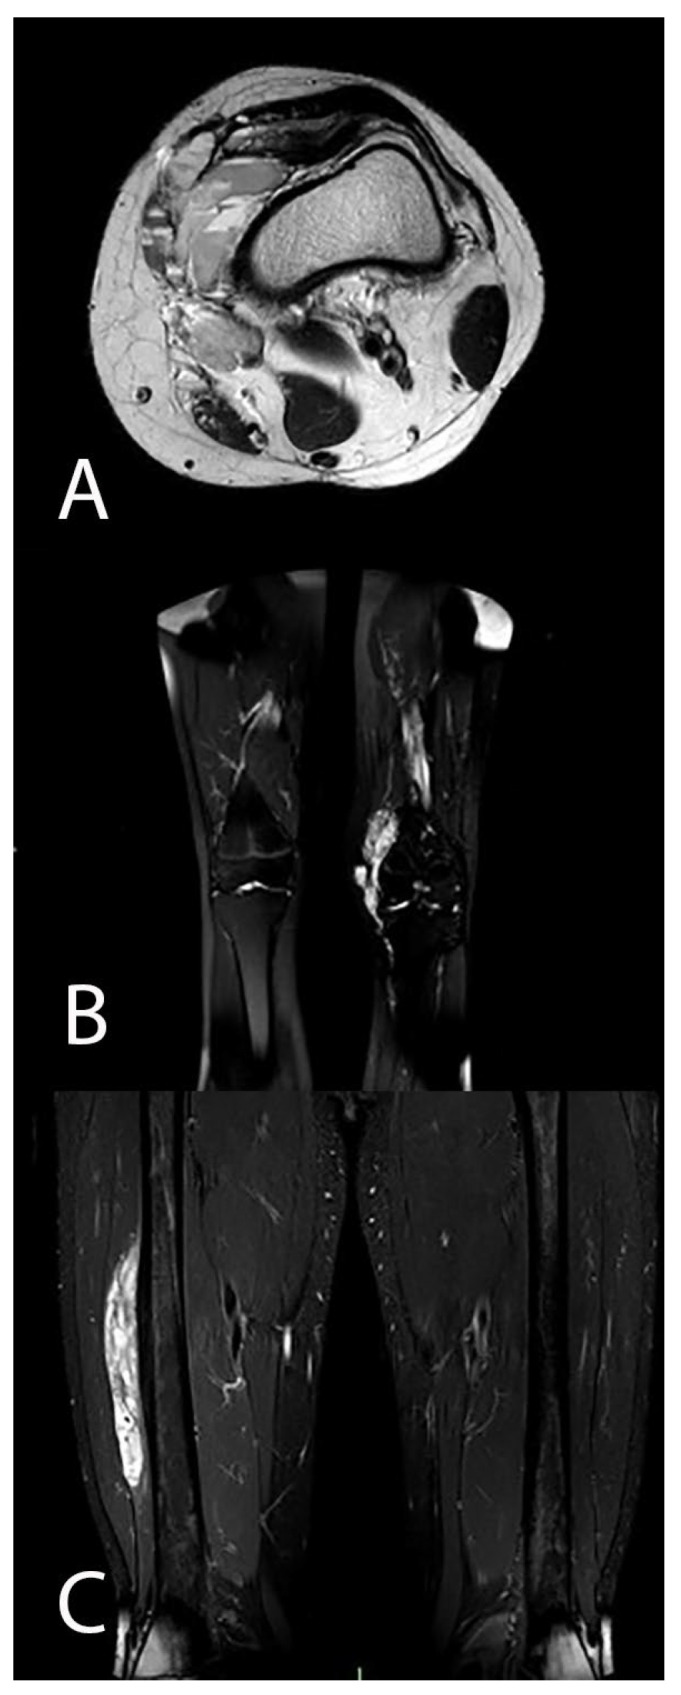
(A). Axial view T2 sequence MRI showing increased signal of a soft tissue MAV near the medial region of the knee, showing some fluid–fluid level related to possible hemorrhage or high protein content. (B). Coronal view T2 fat sat sequence MRI demonstrating hyperintensity of the signal of a soft tissue venous malformation in the medial region of the knee, with some intralesional low-intensity septa. T2 sequences help define the extent of the lesion. (C). Coronal view STIR sequence MRI shows high hyperintensity of the signal of an intramuscular venous malformation adjacent to the femoral diaphysis.
